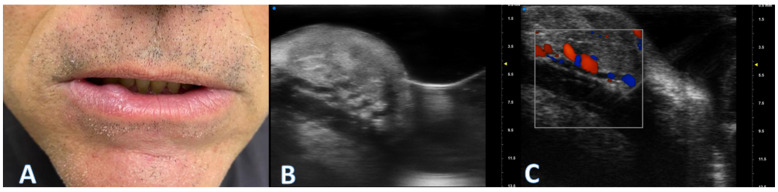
(A) Patient presenting with a round-shaped mildly consistent subepithelial formation. Overlying skin shows no dyschromia or any other significant alteration. (B) The high-frequency ultrasound reveals a well-defined, hyperechoic mass within the oral labial mucosa consistent with an angioleiomyoma. The lesion displays regular borders and appears to be encapsulated and localized within the superficial layers of the mucosa. Vascular structures are visible within the mass as areas of hypoechoic focal inhomogeneity, demonstrating hypervascularity upon Doppler imaging (C). Surrounding tissues exhibit normal echogenicity without signs of infiltration or structural alterations.
